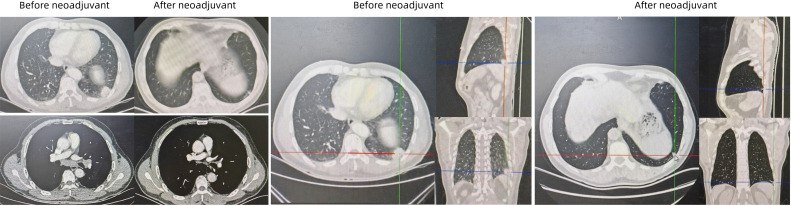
Images before and after neoadjuvant nivolumab immunotherapy combined with nab-paclitaxel and carboplatin chemotherapy. Post-therapy CT showed significant lesion reduction.
